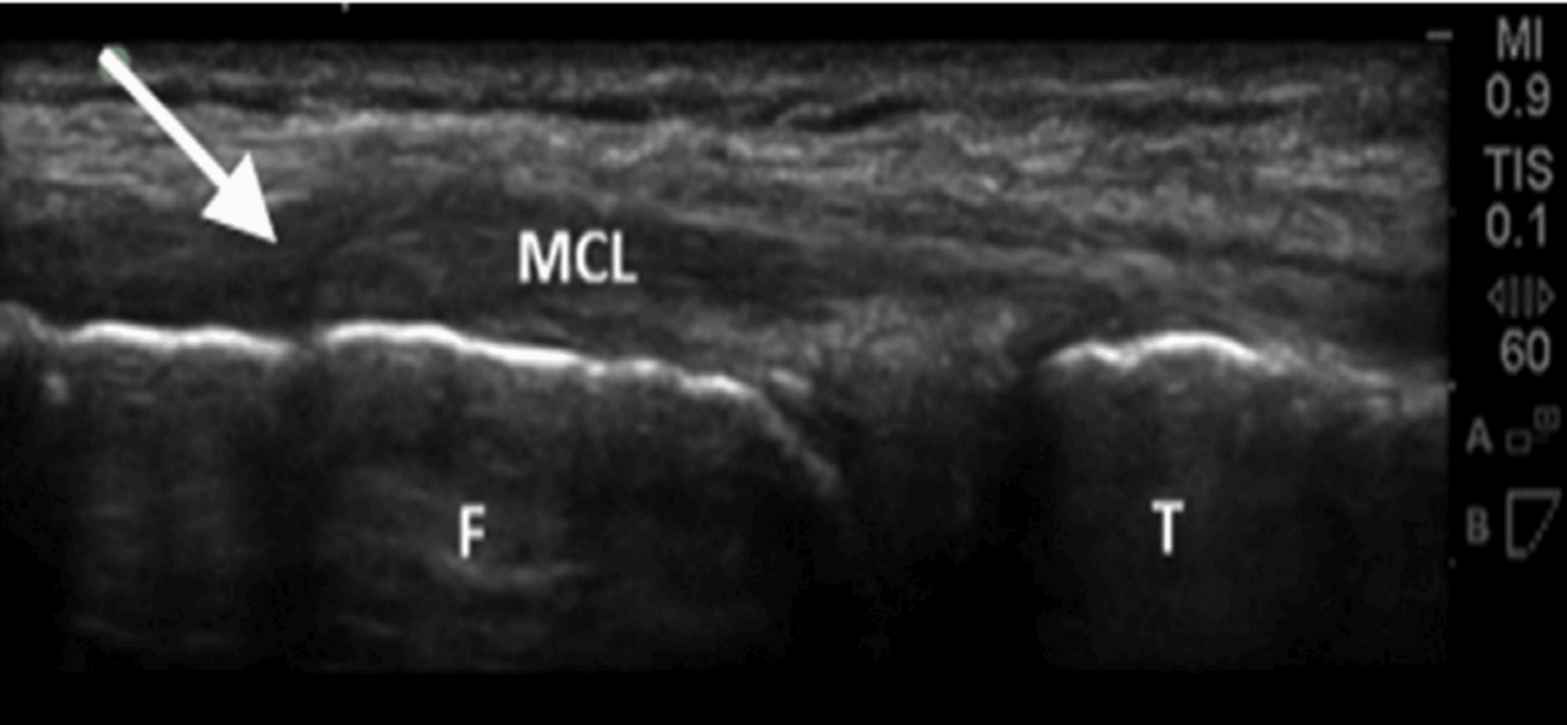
Ultrasound image of proximal MCL tear (arrow), noting ligament tissue disruption with retraction. Both the distal femur (F) and proximal tibia (T) are depicted with the adjoining MCL. The image is published under a Creative Commons License. From Ghosh et al. [14]. MCL: medial collateral ligament
